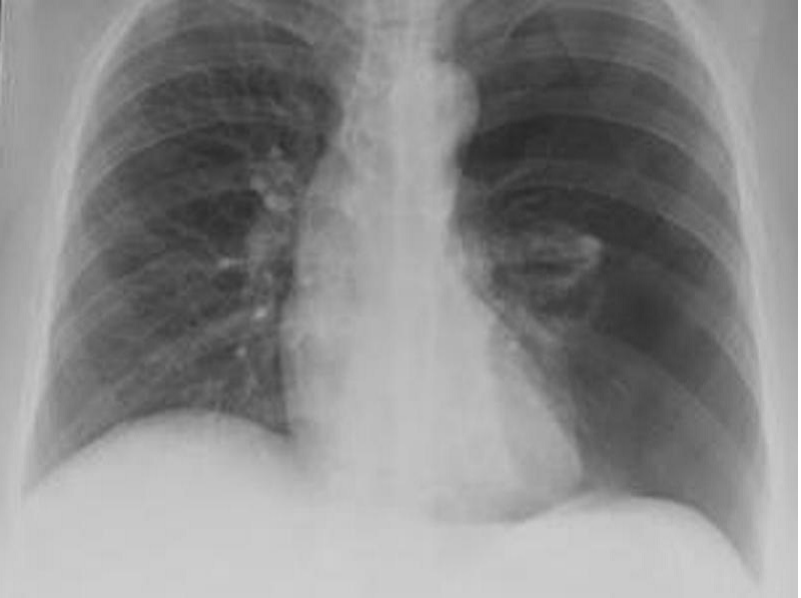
Chest X-ray showing large left pneumothorax with collapsed lung.
